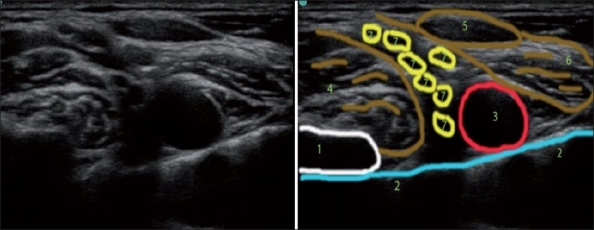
Sagital supraclavicular ultrasound scan. (1) First rib, (2) lung, (3) subclavian artery, (4) middle scalene muscle, (5) omohyoid muscle, (6) anterior scalene muscle, (7) brachial plexus
